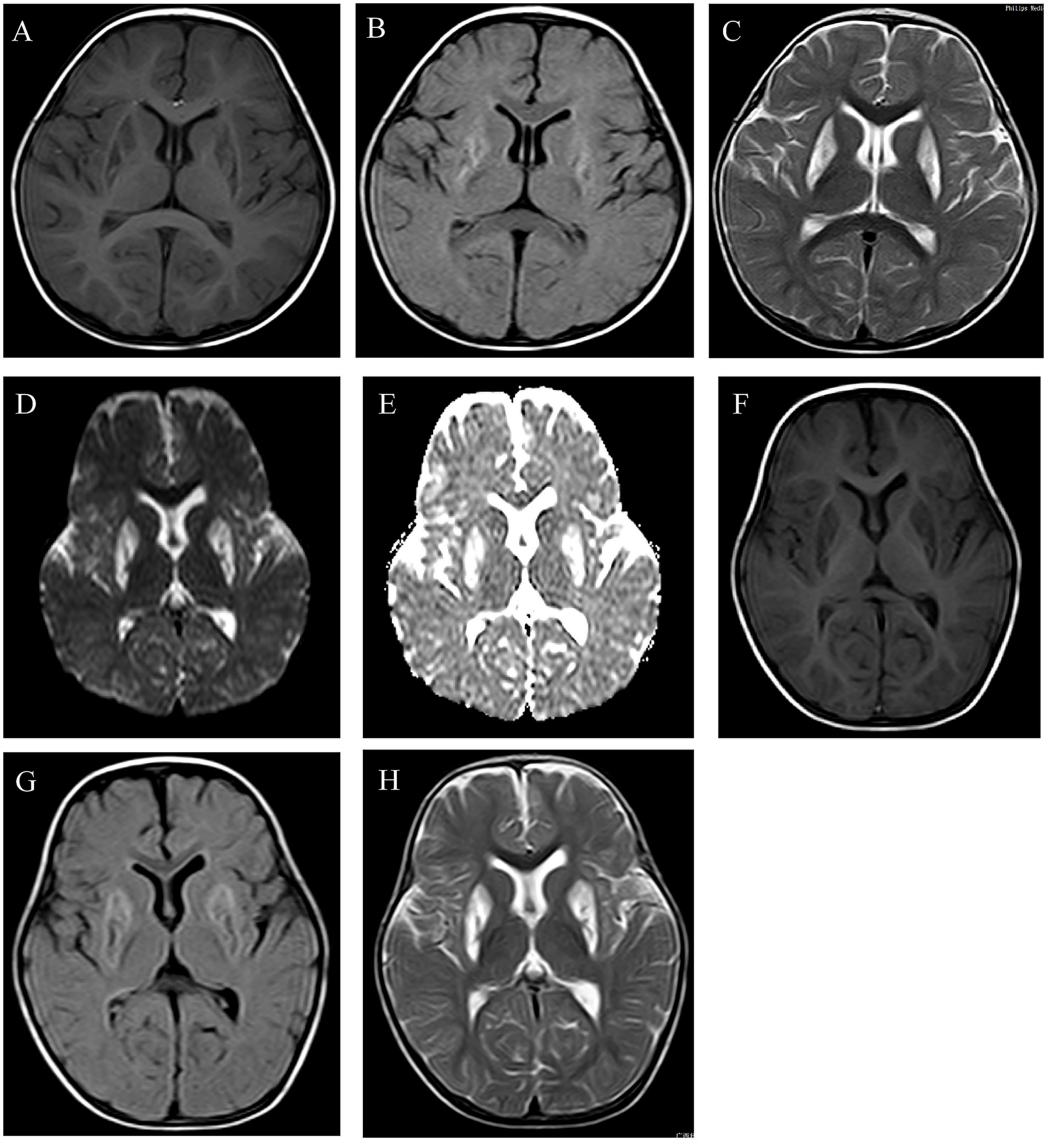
Brain imaging manifestations of patients. MRI images of the patient 1 at 15 months of age (A–C). Symmetrical patchy and small patchy areas of prolonged T1 and T2 signal intensity are observed in both putamen and thalamus. MRI images of the patient 1 at 15 months of age (D–H). Multiple symmetrical abnormal signal lesions are visible in both frontal lobes and basal ganglia regions. These lesions exhibit predominantly high signal intensity on diffusion-weighted imaging (DWI) (D), and low signal intensity on the diffusion coefficient (ADC) map (E), low signal intensity on T1-weighted images (T1WI) (F), predominantly high signal intensity on FLAIR sequences with a minority showing low signal intensity (G), and high signal intensity on T2-weighted images (T2WI) (H).
